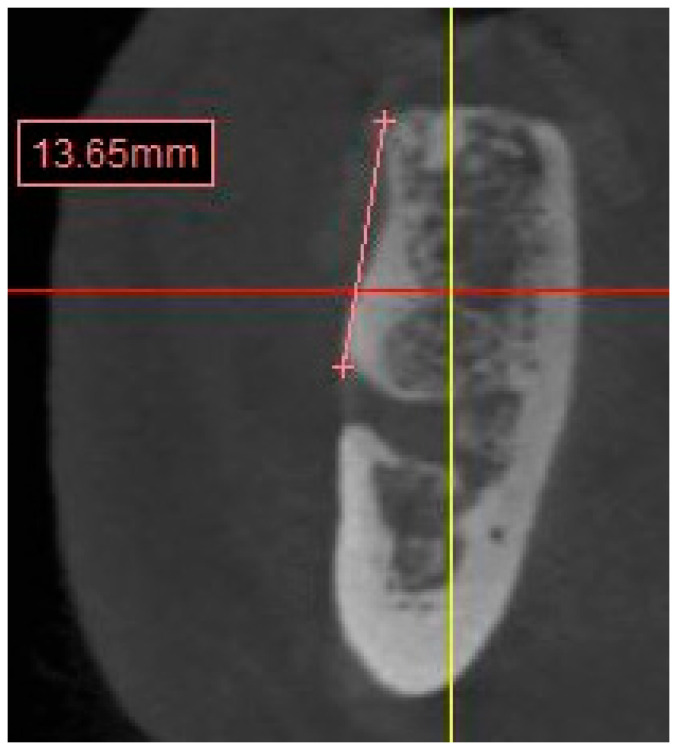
CBCT image showing the measurement calculation for the distance from mandibular ridge to the superior boarder of the mental foramen.
